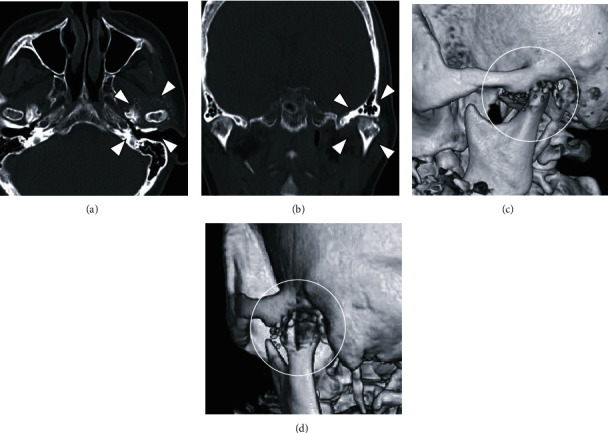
CT images from first examination: (a) axial image, (b) coronal image, and (c, d) 3D image. Deformation of the left mandibular condyle is seen, with many small, granular nodules in the area of the left TMJ, some of which are calcified.
